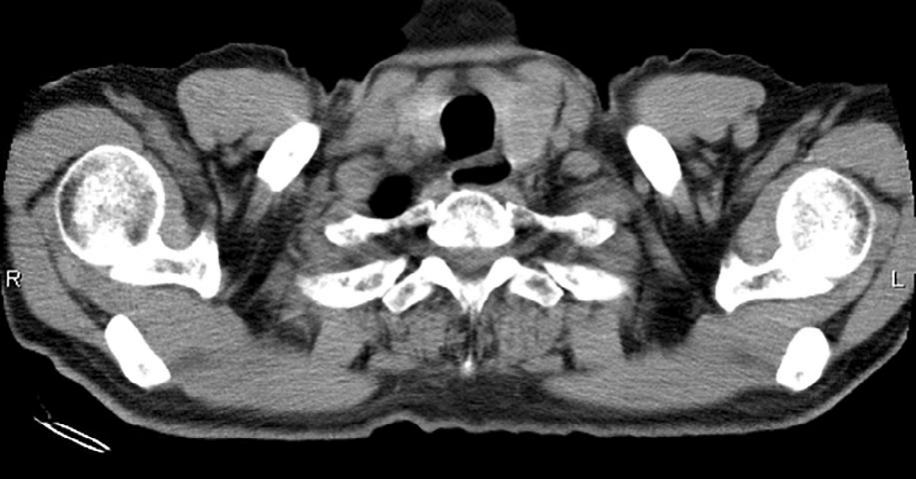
Chest plain computed tomography revealed a round low‐density area in the left thyroid lobe.
